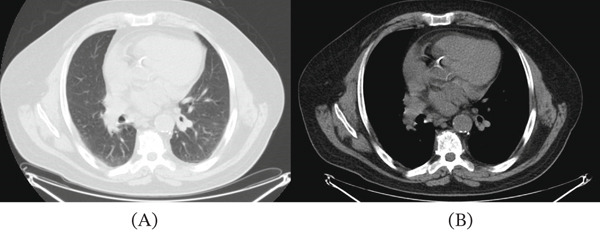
Chest computed tomography (CT). (A) Axial image in lung window showing the hilar region and (B) axial image in mediastinal window demonstrating hilar–mediastinal structures.
